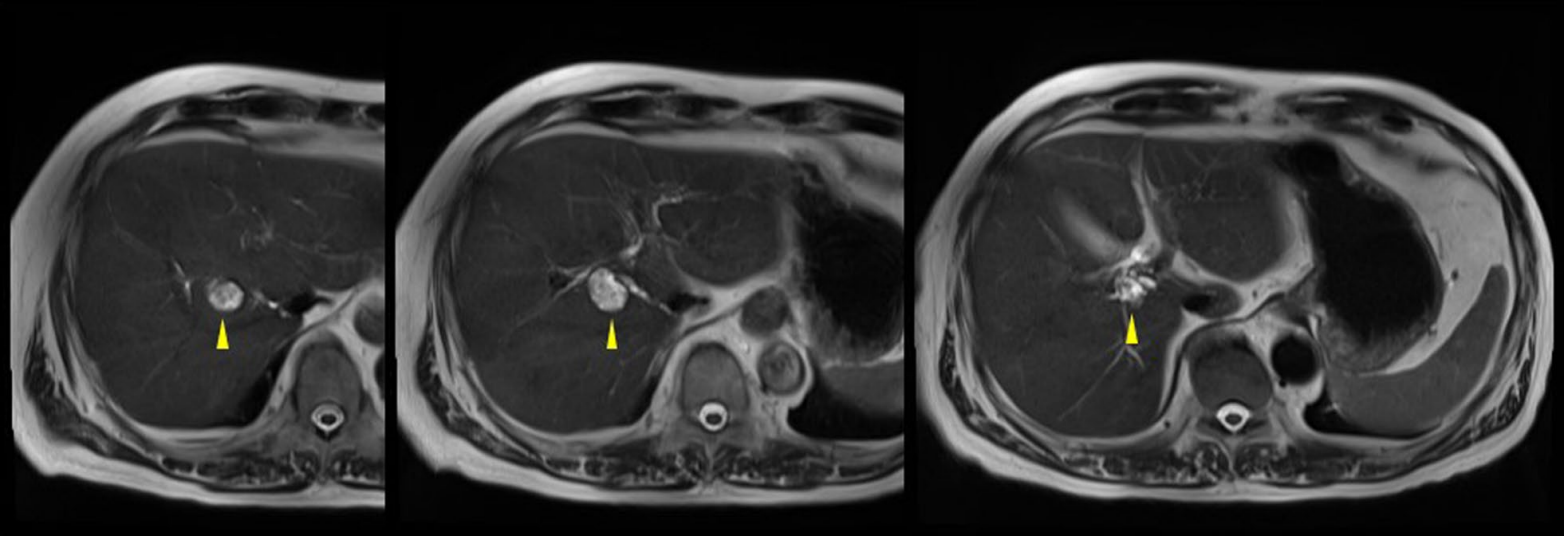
Magnetic resonance imaging. Magnetic resonance imaging showed high intensity on T2 weighted images with a heterogeneous low-intensity area in the lumen (arrowhead)
